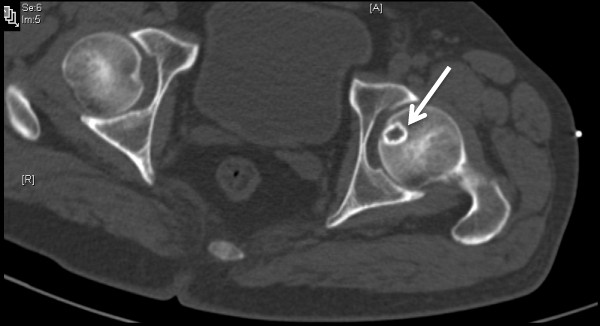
Axial computed tomography section confirming a clearly defined lucent nidus with surrounding sclerotic rim (white arrow).
